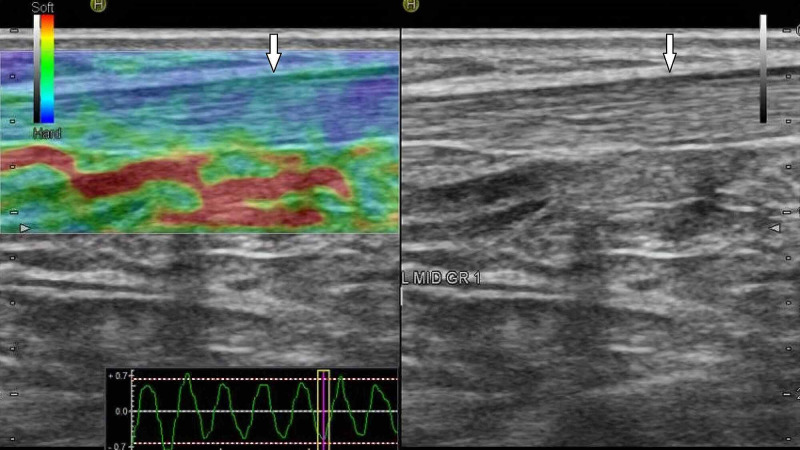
Normally appearing grade 1 Achilles tendon. B-mode (right image) and sonoelastographic (left image) ultrasound images of the left middle part of Achilles tendon (arrows) in a 21-year-old woman with familial Mediterranean fever (FMF).
